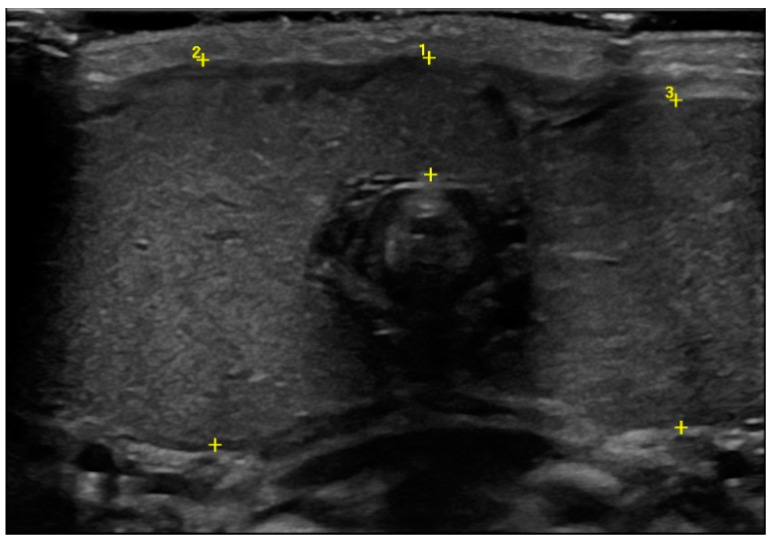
Thyroid ultrasound at birth in which the enlargement of the gland was detected (cursor 1 isthmus, 0.8 cm; cursor 2 antero–posterior diameter of the right lobe, 2.5 cm; cursor 3 antero–posterior diameter of the left lobe, 2.2 cm).
